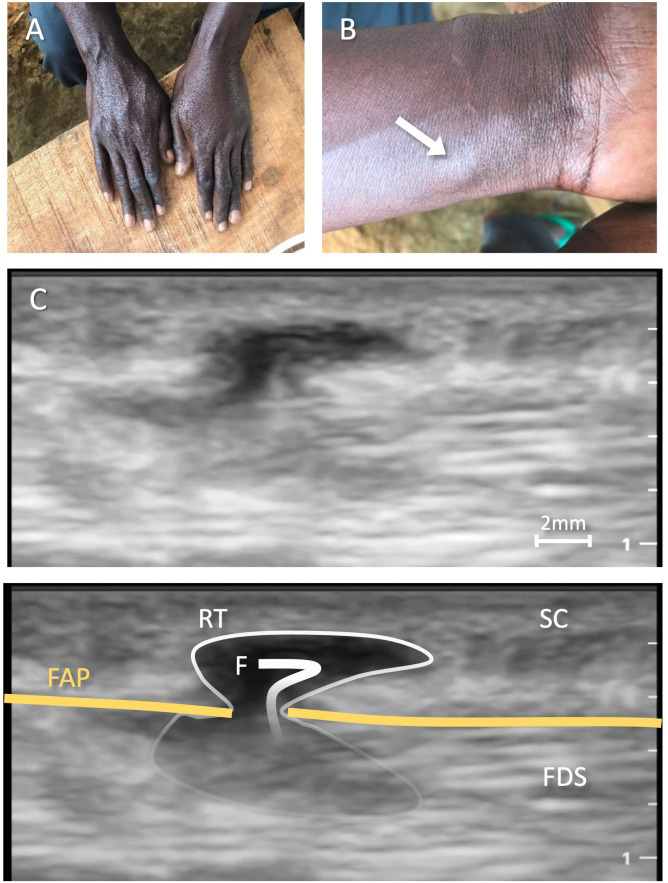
Findings of Patient 5: A) Typical Calabar swelling on the left hand; B) Subcutaneous nodule on the volar aspect of the left distal forearm; C) Corresponding ultrasound image revealing a subcutaneous, anechoic lesion presenting as retention, with trans-fascial gap and hyperechoic structure inside the retention. The thread like structure is less than 0.5 mm in diameter (compare to the 2 mm scale bar, still image from S2 Videos at second 14); D) Corresponding ultrasound image with annotations. Legend: RT = retention; F = filaria; SC = subcutaneous tissue; FAP = *palmar antebrachial fascia* (orange); FDS = *flexor digitorum superficialis muscle*. Pictures A and B were taken by LV.
